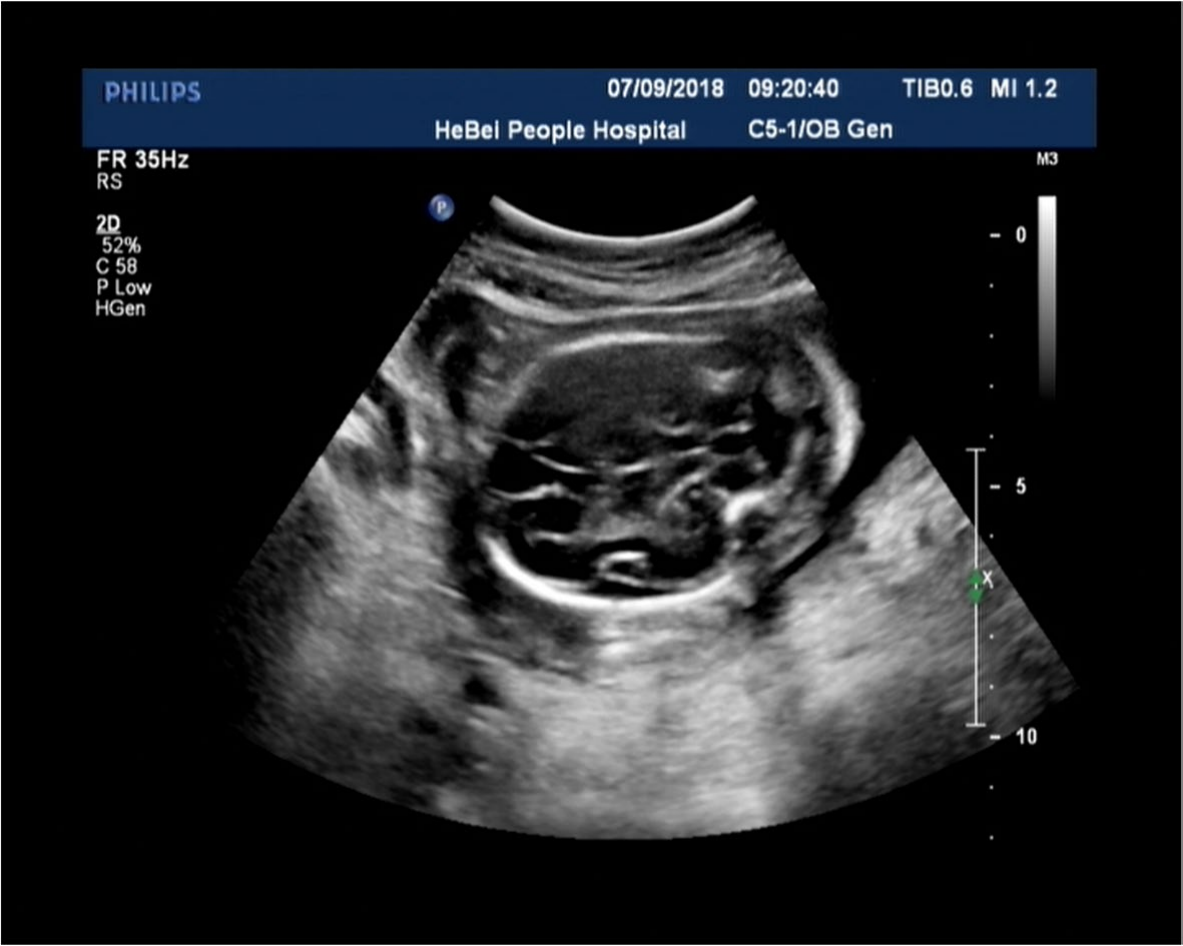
The ultrasound example of a DWS foetus. It shows a partial absence of the vermis, dilation of the fourth ventricle, and an enlarged posterior fossa
